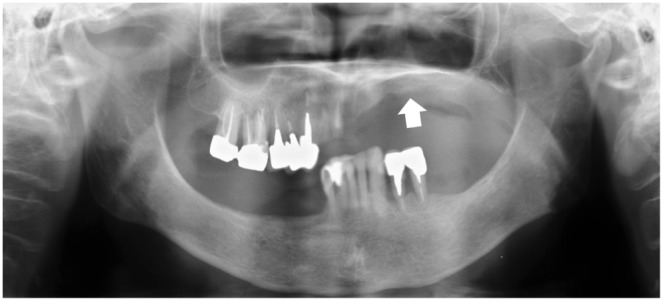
Typical panorama x-ray view of Eichner Index B4 patient. Note the absence of posterior occlusal support with highly resorbed alveolar bone (white arrow).
